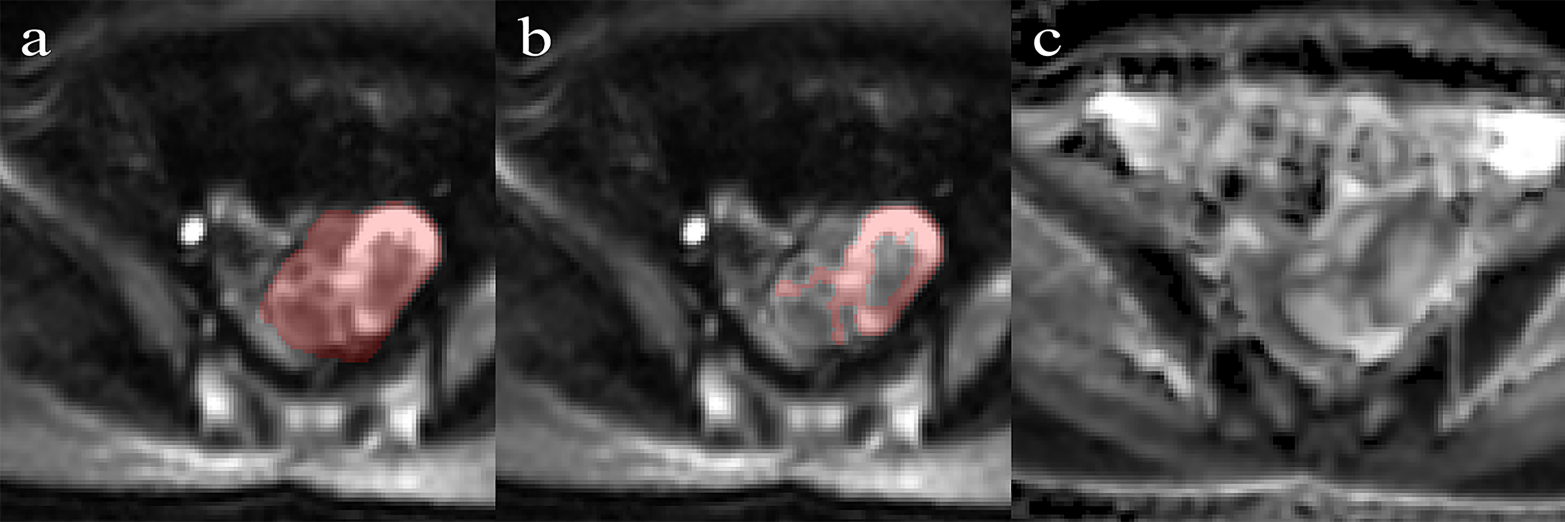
Performance of region of interest on the DWI (*b* = 1000 s/mm^2^) by referring to T2WI. (A) Whole-tumor ROIs are manually drawn along the edge of the tumor on the DWI. (B) Solid-tumor ROIs are drawn along the solid components of the tumor. (C) The corresponding ADC map.
